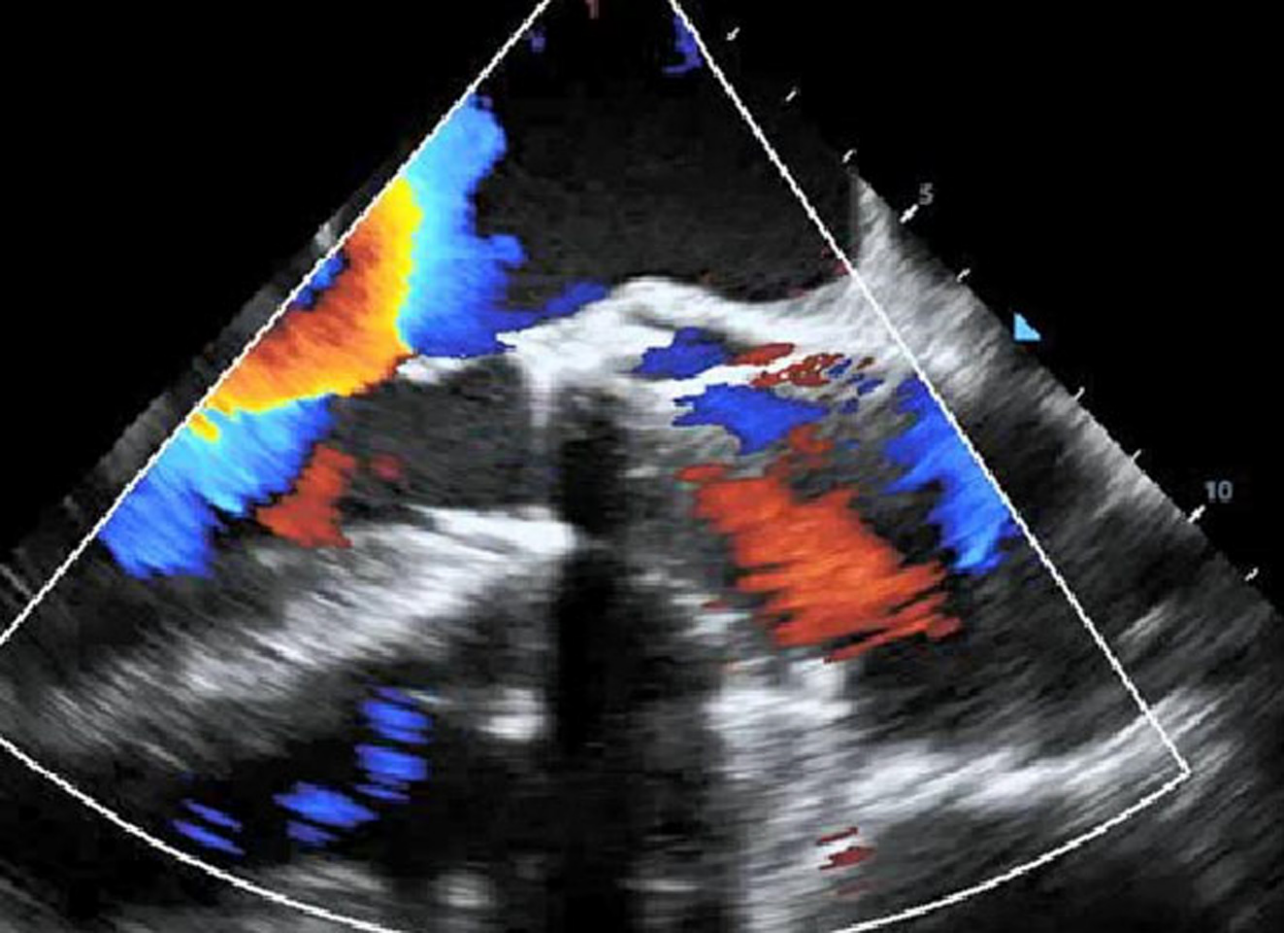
Transesophageal echocardiography After Redo‐TAVR.
